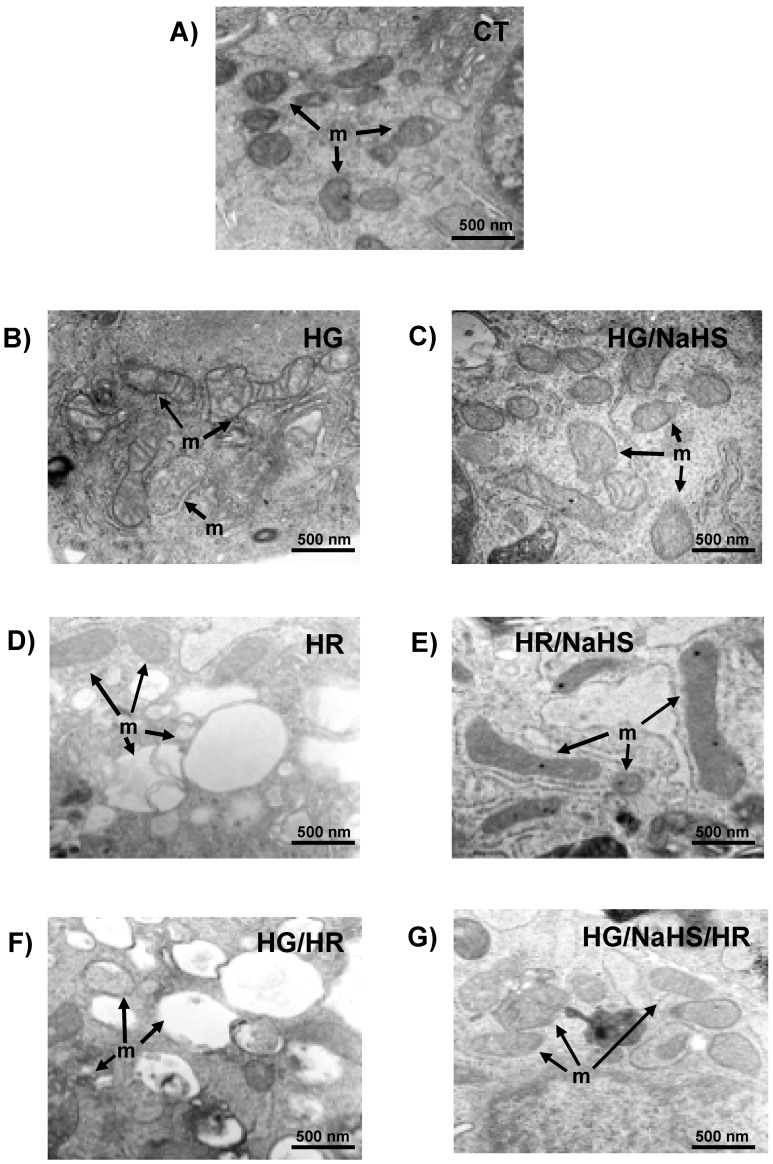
Evaluation of cardiomyocyte ultrastructure in cells incubated with NaHS (100 μM), exposed to HG, HR, or both conditions (HG/HR). NaHS treatment protects cardiomyocytes subjected to HG, HR, or both conditions from mitochondrial ultrastructure damage. (A) CT, (B) HG, (C) HG/NaHS (100 μM), (D) HR, (E) HR/NaHS (100 μM), (F) HG/HR, and (G) HG/HR/NaHS (100 μM). Arrows indicate mitochondria structures.
